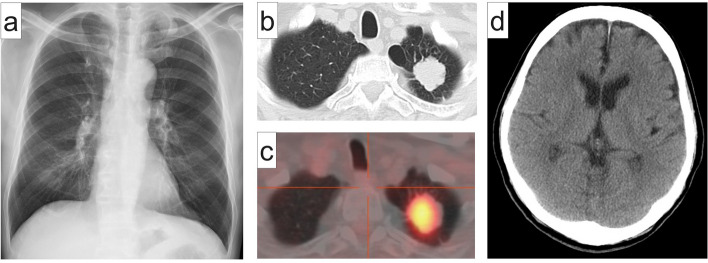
Preoperative imaging. a Chest radiograph shows an abnormal shadow in the left upper lung field. b Chest computed tomography image reveals a 31-mm solid nodule in the left upper lung lobe (S1+2). c Increased 18F-fluorodeoxyglucose uptake is seen within the nodule in the left upper lung lobe. d Brain contrast-enhanced computed tomography image reveals no sign of brain metastasis
